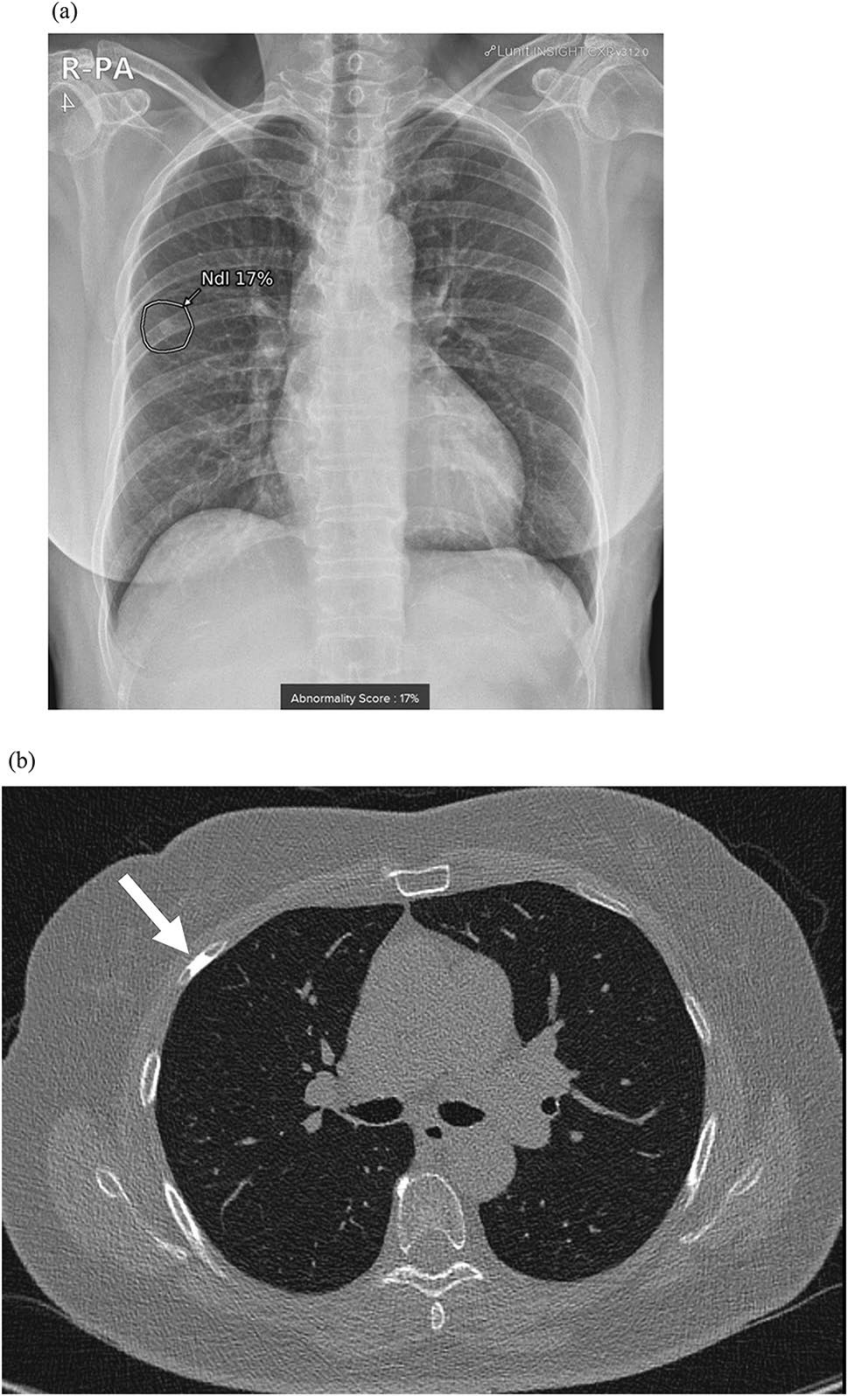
A false positive case of a 71-year-old woman who underwent initial CXR after complaining of headache and hypertension. A nodule detected by AI-software was proven to be a bony island of the right fourth rib. (a) AI-software detects a small nodule (arrow) in the right middle lung field with an abnormality score of 17% on CXR. (b) Corresponding low-dose CT revealed a small homogeneously sclerotic bone lesion within the medullary space of the right fourth rib (arrow), which would be a bony island.
